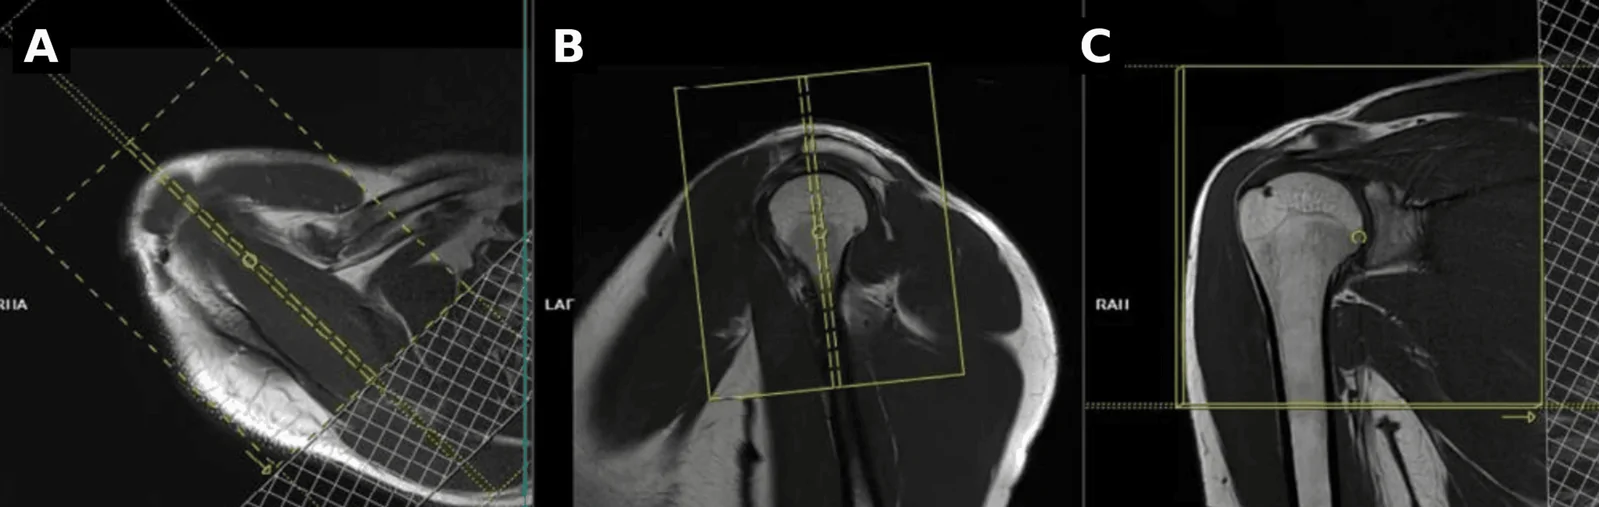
MRI shoulder protocol planning A: axial plane planning image used for evaluation of the subscapularis tendon, long head of biceps tendon, glenohumeral joint, labrum, and anterior/posterior periarticular structures; B: sagittal oblique plane planning image used for evaluation of rotator cuff muscles, muscle bulk, fatty infiltration, tendon orientation, and overall cuff morphology; and C: coronal oblique plane planning image used for evaluation of the supraspinatus and infraspinatus tendons, tendon footprint, subacromial-subdeltoid bursa, and acromioclavicular joint region.
